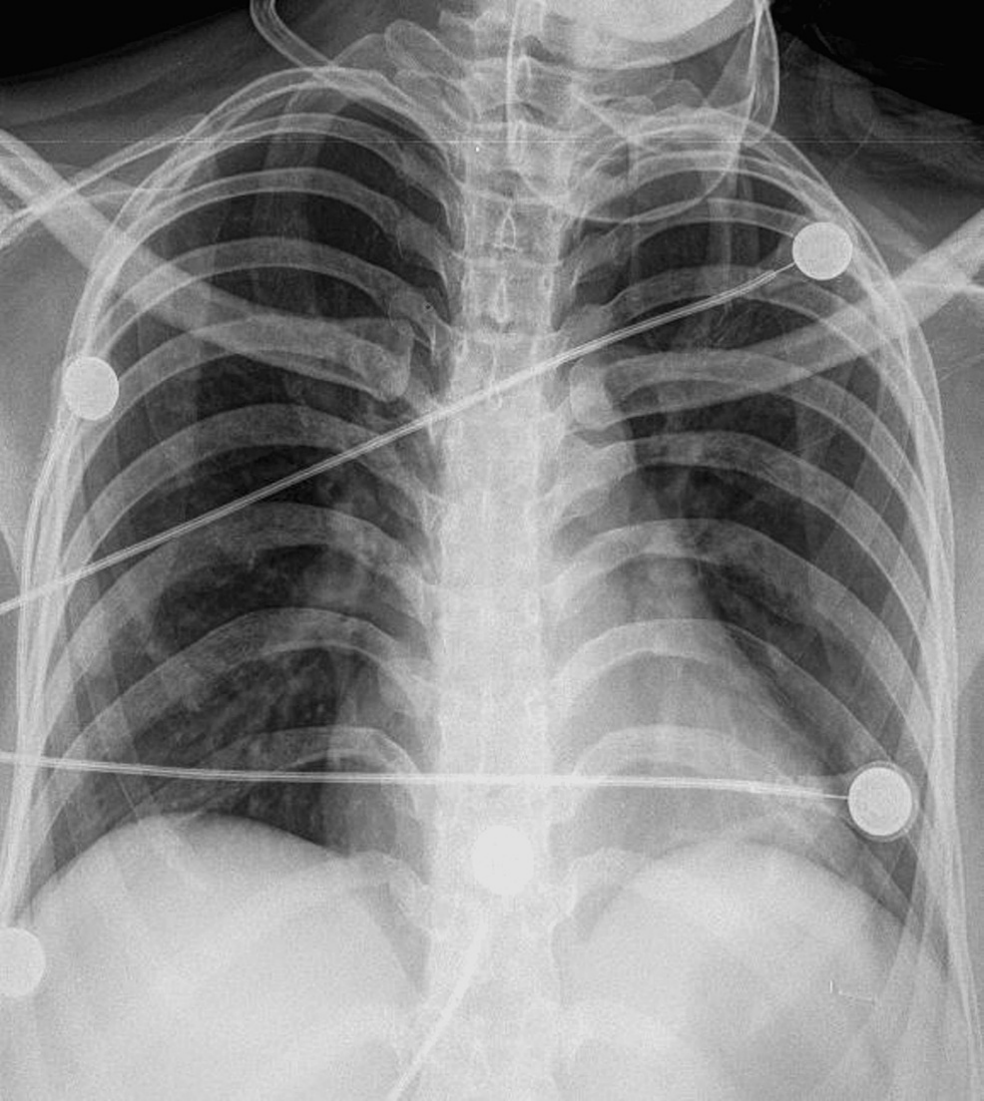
Chest X-ray does not show air under the diaphragm.
